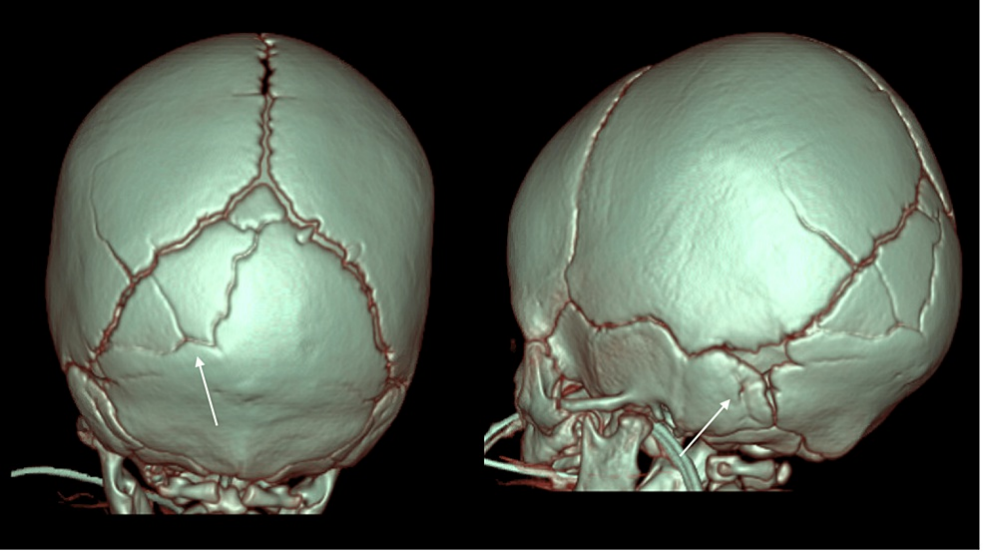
3D CT scan findings of accessory cranial suture with concomitant TBI in a 4-month-old male coronal (left) and sagittal (right) views. There are no calvarial fractures; there is a linear lucency in the left parietal bone, which is felt to represent an accessory intraparietal suture; and there are wormian bones in the occiput. The coronal and sagittal sutures are patent. The lambdoid sutures are patent.
